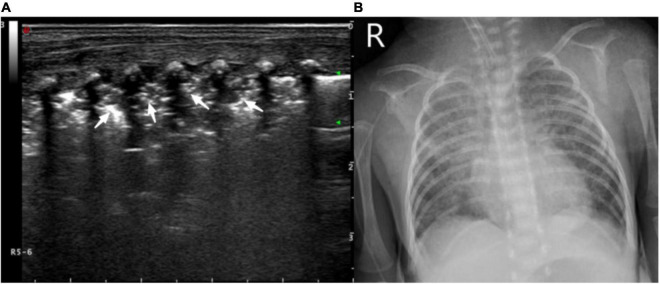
(A) Ultrasound manifestations of large-area consolidation of newborn lungs. Pulmonary ultrasound shows a large irregular consolidation area with air bronchogram (white arrow) and the disappearance of the pleural line and A-line. The disappearance of the pulmonary slip and lung pulse can be observed with real-time ultrasound. (B) X-ray showing a diffuse patchy fuzzy shadow of both lungs and a bronchogram of lower field.
